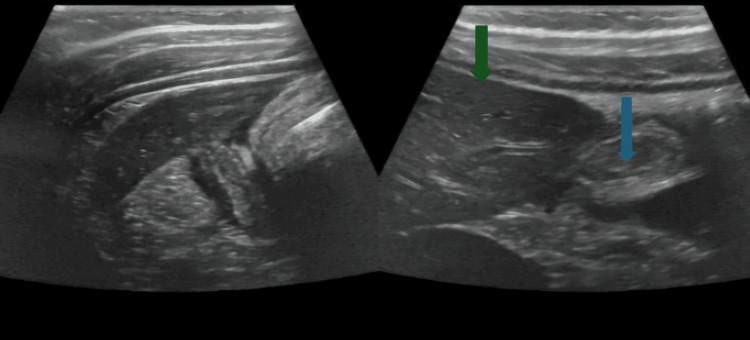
Ultrasound showing subhepatic appendix Blue arrow: Subhepatic appendix, Green arrow: Liver
